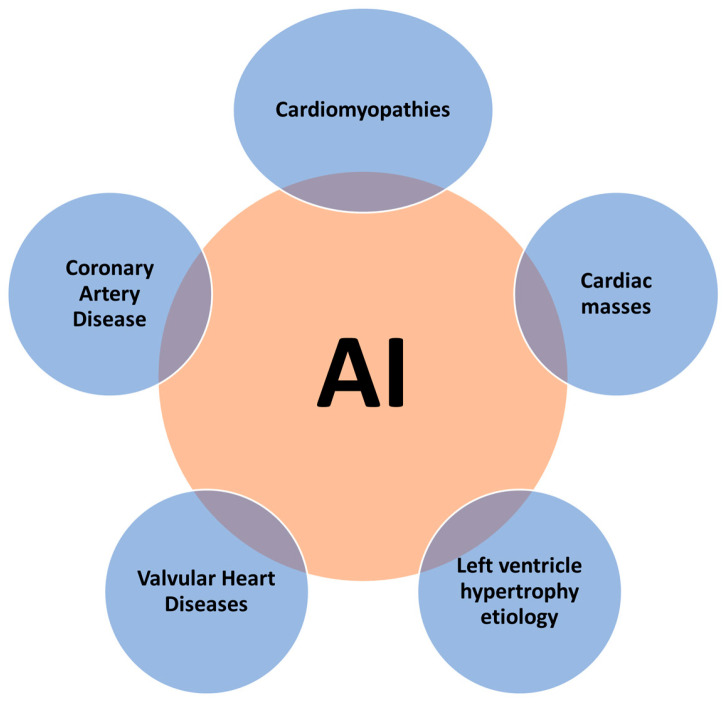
Potential clinical applications of artificial intelligence in echocardiography to identify disease states.
